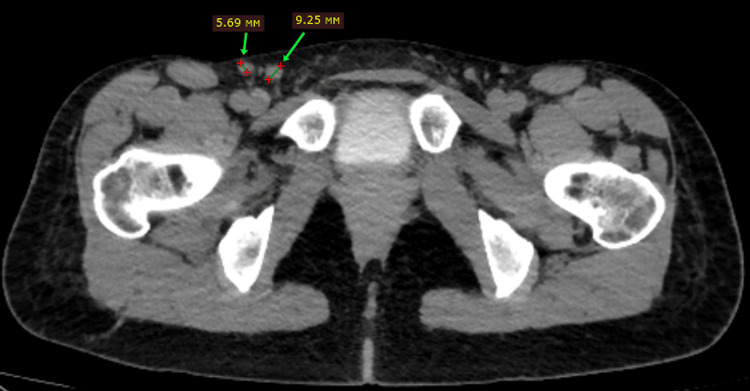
Computed tomography after neoadjuvant chemotherapy demonstrating the regression of the inguinal lymph nodes Axial computed tomography image obtained after four cycles of neoadjuvant chemotherapy with carboplatin and paclitaxel demonstrating the regression of previously enlarged right inguinal lymph nodes.
